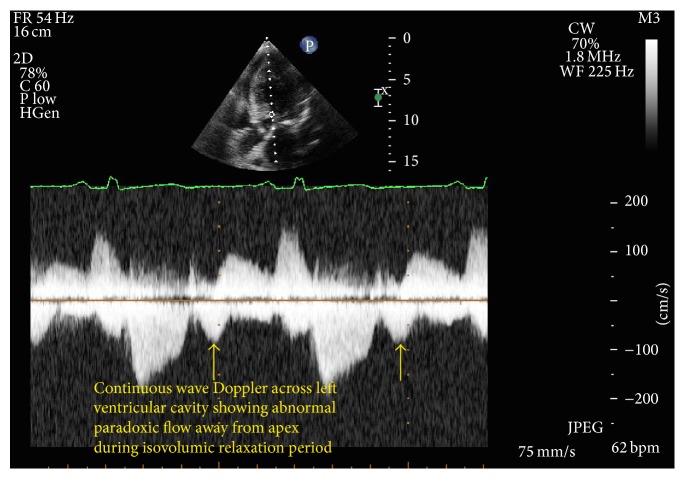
Doppler flow pattern across midventricular obstructive ventricle.
